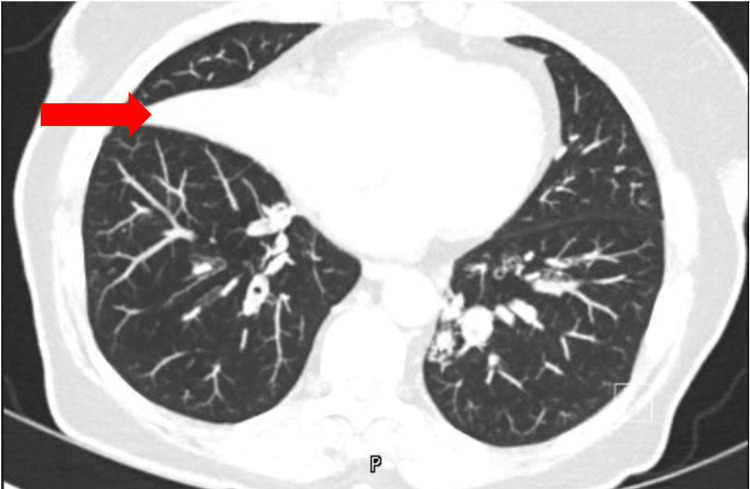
Axial plane of chest CT showing complete atelectasis of the right middle lobe and bronchiectasis with severe mucoid impaction in the left lower lobe.
